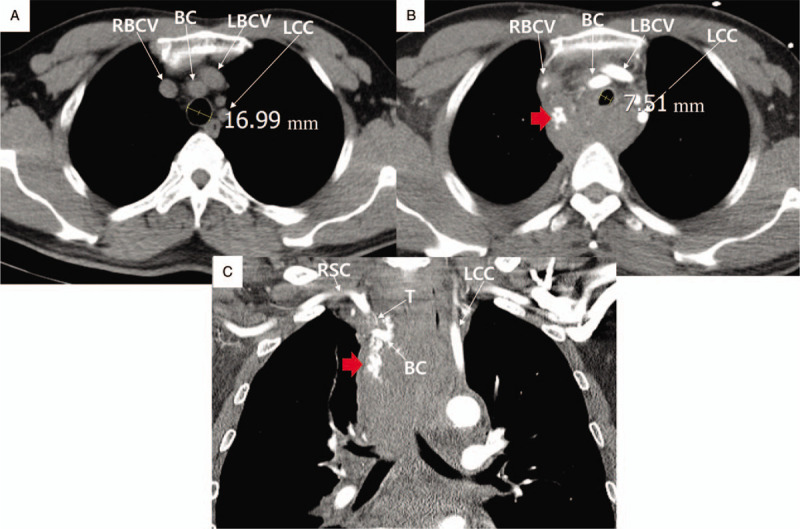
Chest computed tomography. A. The pre-interventional image (1 year ago) shows the normal range of mediastinum, trachea, and lung. B. The post-interventional image after coronary stenting shows mediastinal hematoma, tracheal compression, and hemothorax, suggesting brachiocephalic arterial rupture. C. The post-interventional coronal section image after coronary stenting shows the extravasated blood around the brachiocephalic trunk region and a large mediastinal hematoma. A red arrow indicates the active bleeding site. The numbers indicate the tracheal diameters. BC = brachiocephalic artery, LBCV = left brachiocephalic vein, LCC = left common carotid artery, RBCV = right brachiocephalic vein, T = right inferior thyroid artery.
